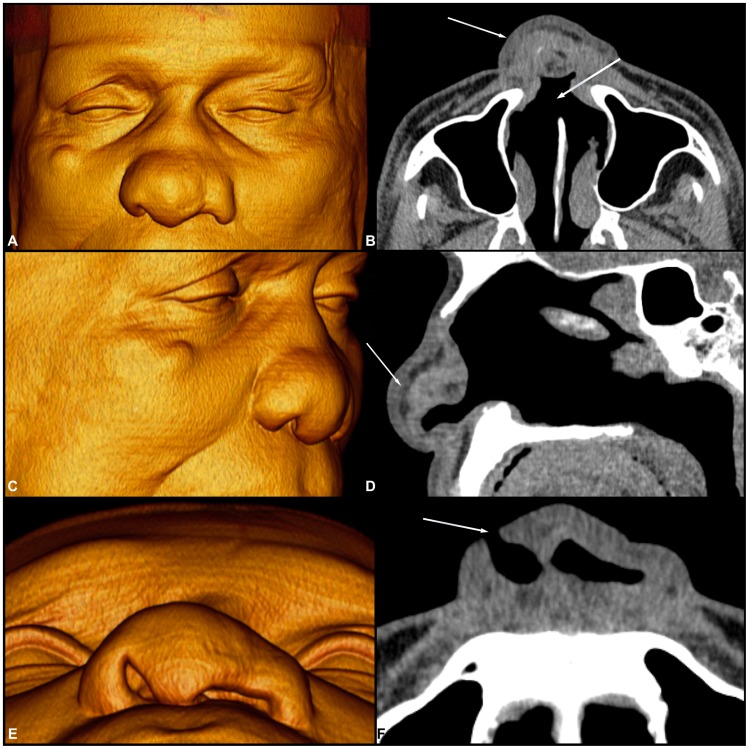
Volume-rendered 3D images of multi-slice CT data (A, C and E), axial CT scans (B and F) and a sagittal CT scan (D) with a soft tissue window showing diffuse thickening of the nasal wings and collapse of the nasal pyramid. Axial CT scan shows the erosion of the nasal septum (B and D) associated with diffuse thickening of the nasal wings and collapse of the nasal pyramid (B, D and F).
